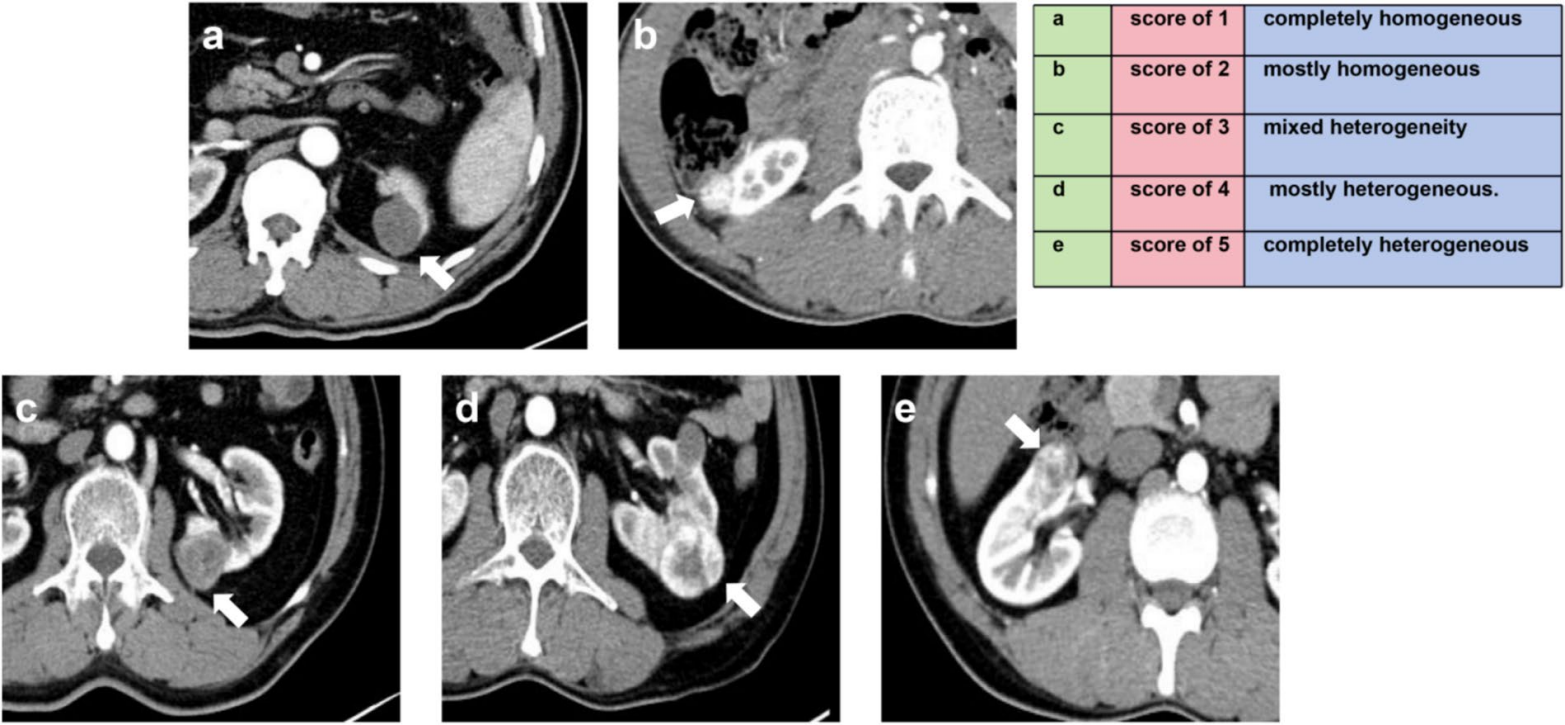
Examples of assessing the 5 grades of homogeneity score based on CT images in axial corticomedullary phase
